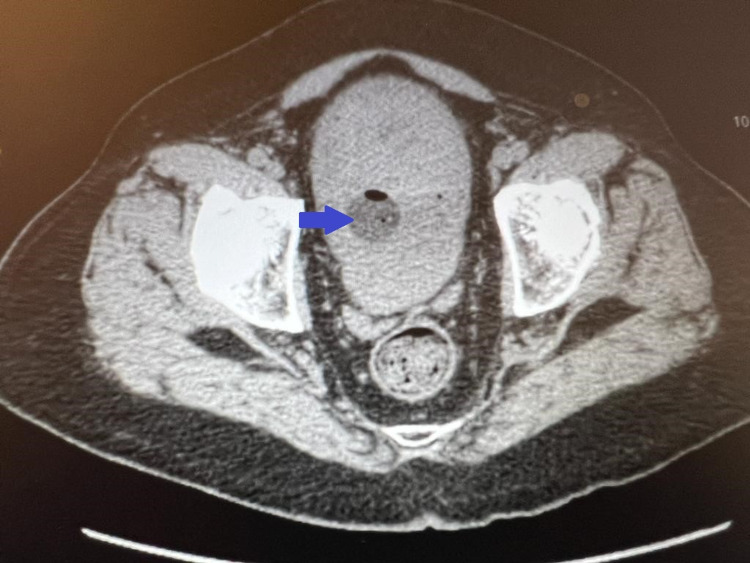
Computed tomography (CT) of abdomen and pelvis showing a large filling defect within the urinary bladder suspicious for a blood clot (blue arrow)
